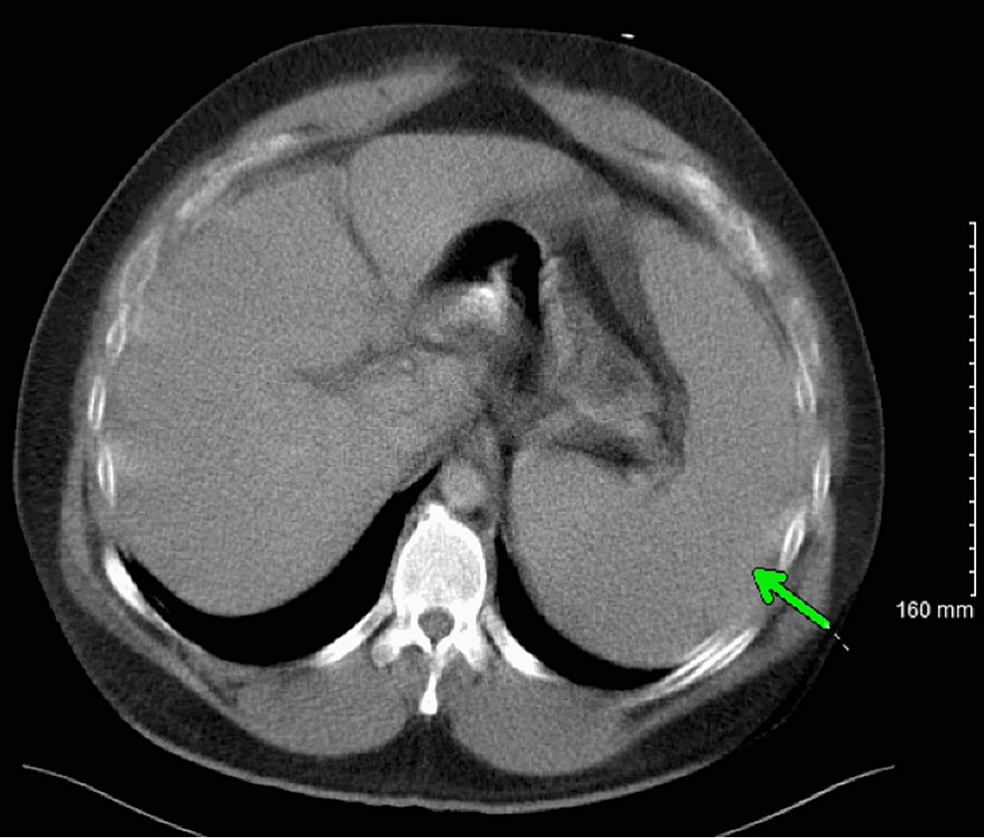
Computed tomography (CT) of the abdomen, with axial view showing (green arrow) splenomegaly
